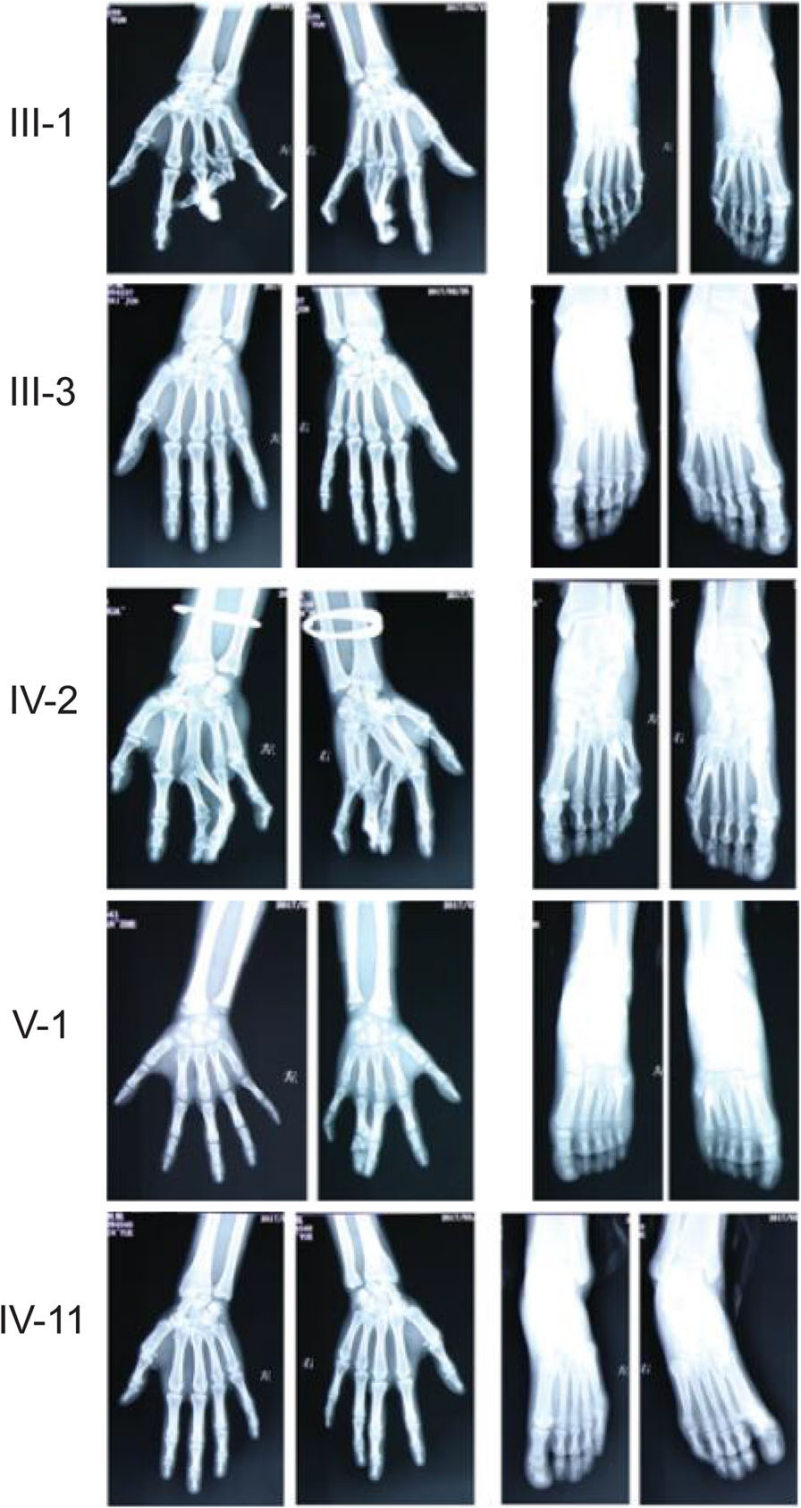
Radiographs of the affected members of the family in which hand and foot deformities are clearly visible
